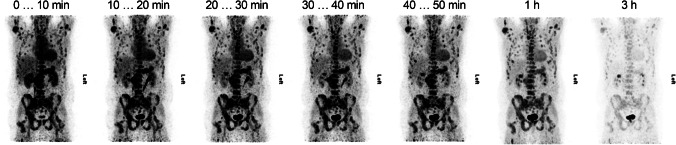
Maximum-intensity-projections of the PET images of a patient (Pat. 6) from the base of the skull to the mid-thigh including whole-body CT imaging. The first 6 PETs were taken within the first 1 h, starting with the injection and covering a time span of 10 min each. Prior to this series the corresponding auxiliary CT was acquired. The seventh PET was started after 3 h, with an acquisition time of 2.5 min per bed. For this series, a separate auxiliary CT was acquired
